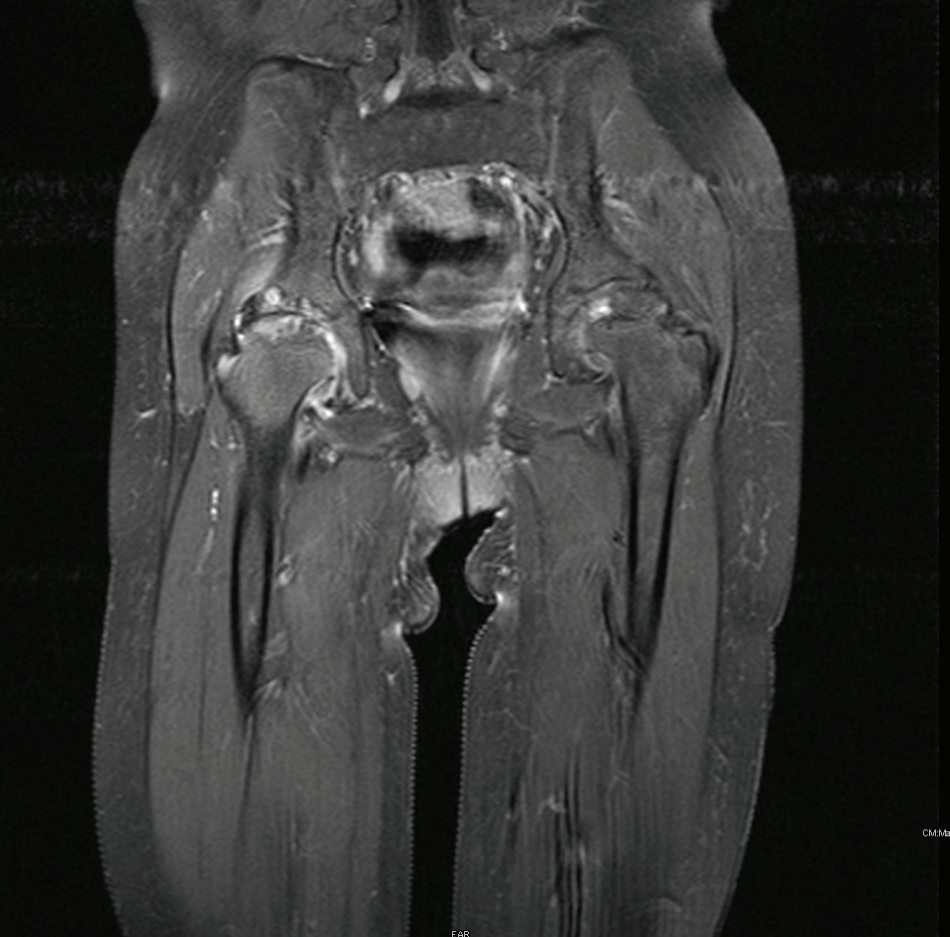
MRI Scan of the Patient. MRI with gadolinium showing active inflammation with synovial thickening, cortical irregularity, loss of cartilage and subcortical oedema on the right hip joint and chronic changes on the left hip joint.
